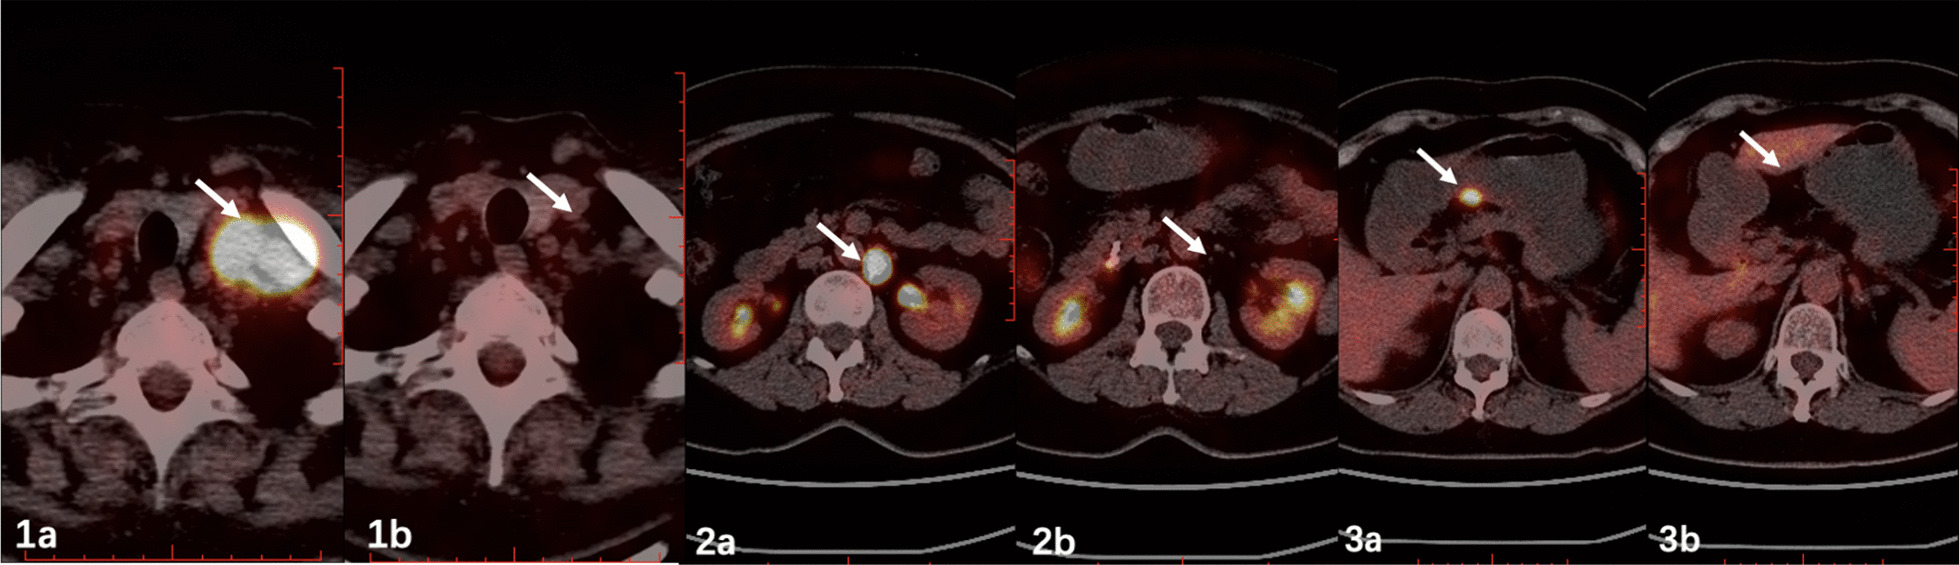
PET/CT revealed lymph nodes with increased metabolism at the bilateral supraclavicular fossa, para- abdominal aorta and lesser curvature of stomach on admission (Fig. 1b, 2b,3b) were no longer as obvious as 3 years ago (Fig. 1a, 2a,3a)
